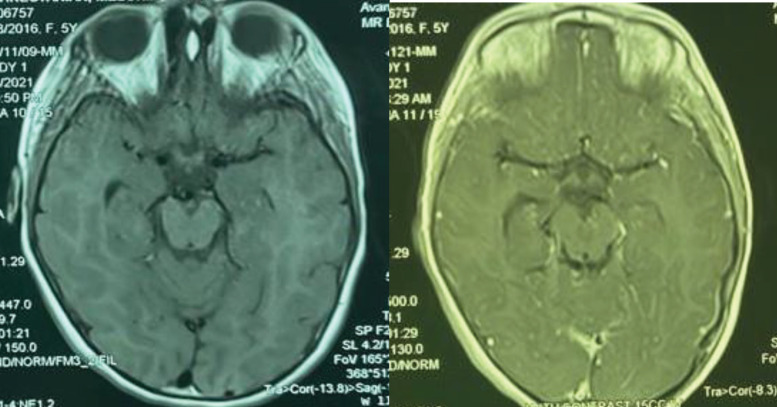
Brain MRI upon admission
